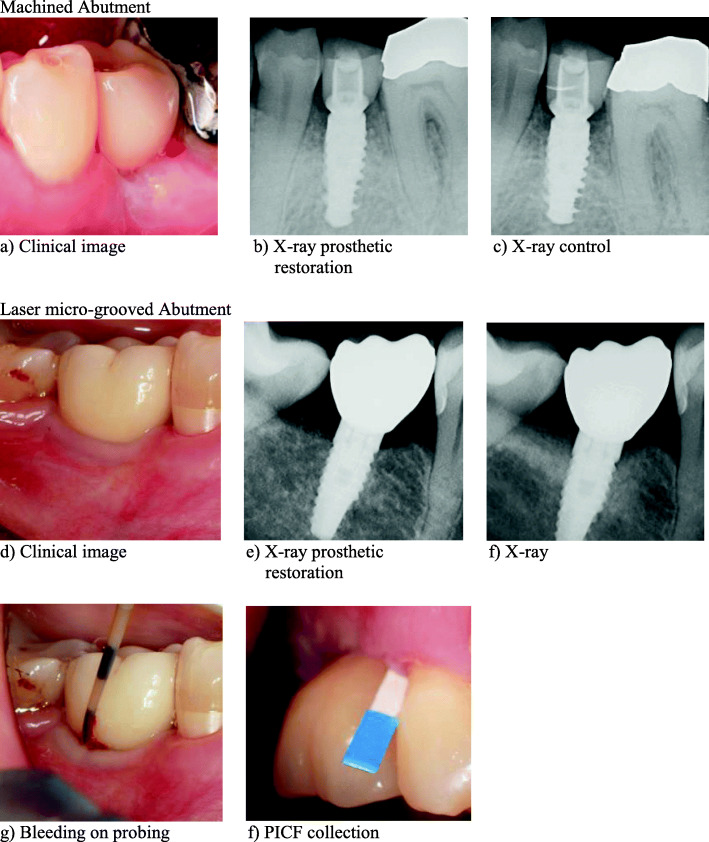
Clinical and radiographic images. Machined abutment: a clinical image, b X-ray prosthetic, and c X-ray control restoration. Laser microgrooved abutment: d clinical image, e X-ray prosthetic, f X-ray restoration, g bleeding on probing, and f PICF collection
